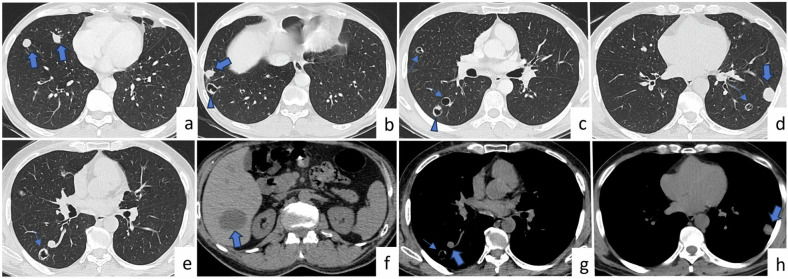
Pulmonary hydatid cyst. A 58-year-old patient with no known disease is admitted to the hospital with cough, fever, and malaise. Multiple nodules are seen at different locations on axial (a–e) chest CT images. These nodules have variable sizes and shapes. On axial chest CT images (mediastinal window) (g, h), these nodules are seen as low density (thick arrows). There is also a hypodense lesion in the liver on abdominal CT images (f, thick arrow). Some of the lesions are completely ruptured, and some of them represent contained rupture (b–e, thin arrows and arrowheads). Mass within cavity sign (b, c, arrowheads), and empty cyst sign (c–e, g, thin arrows) are complete rupture signs of pulmonary HC. Echinococcus IgG was positive
